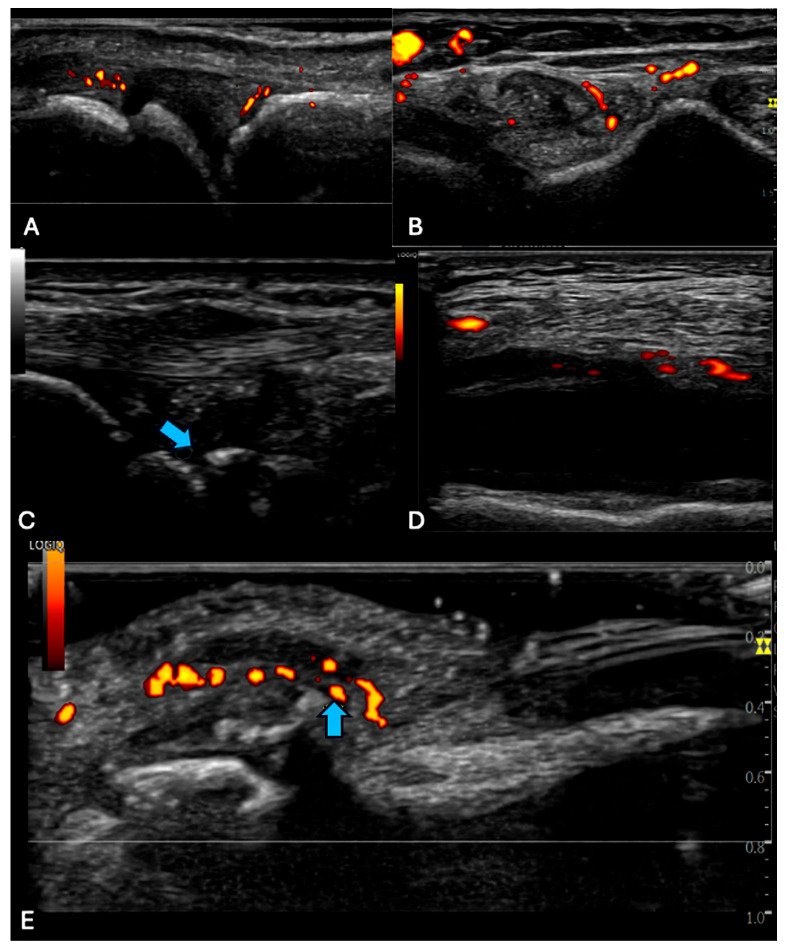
Representative ultrasound images showing (A) MCP synovitis, long axis, (B) tenosynovitis of the wrist, short axis, (C) erosion of the lunate, wrist, long axis (arrow), (D) transverse suprapatellar recess with effusion, synovial hypertrophy, and power Doppler, short axis, and (E) enthesitis DIP extensor tendon, long axis (arrow).
